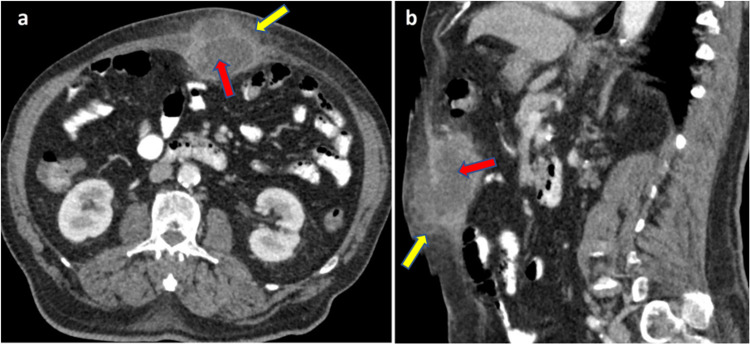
CT of abdomen with IV contrast, a) axial and b) sagittal reformatted image showing a large ill-defined complex hypodense lesion with irregular enhancing wall in supra-umbilical anterior abdominal wall in midline (red arrows) and associated surrounding fat stranding (yellow arrows).
